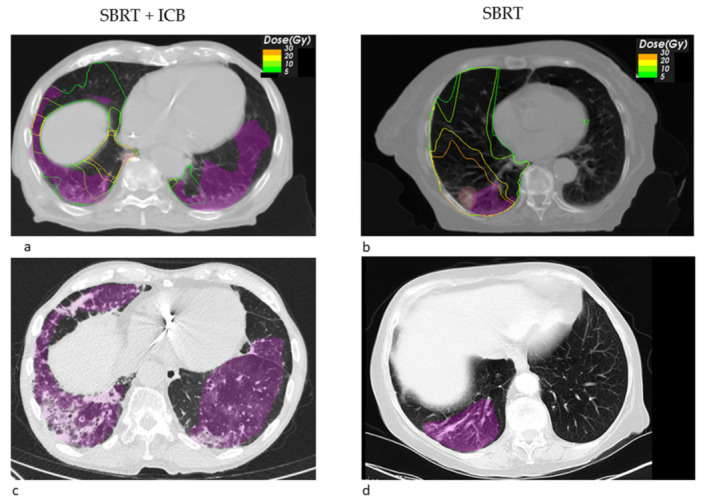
Transversal radiotherapy (RT) planning CT slices showing the EQD2 isodoses and pneumonitis contours (magenta) and GTV contours (light red) for a SBRT + ICB case (a) and for a SBRT case (b). Exemplary transversal slices from the follow up CT showing the pneumonitis and its contours (magenta) for the SBRT + ICB case (c) and the SBRT case (d).
